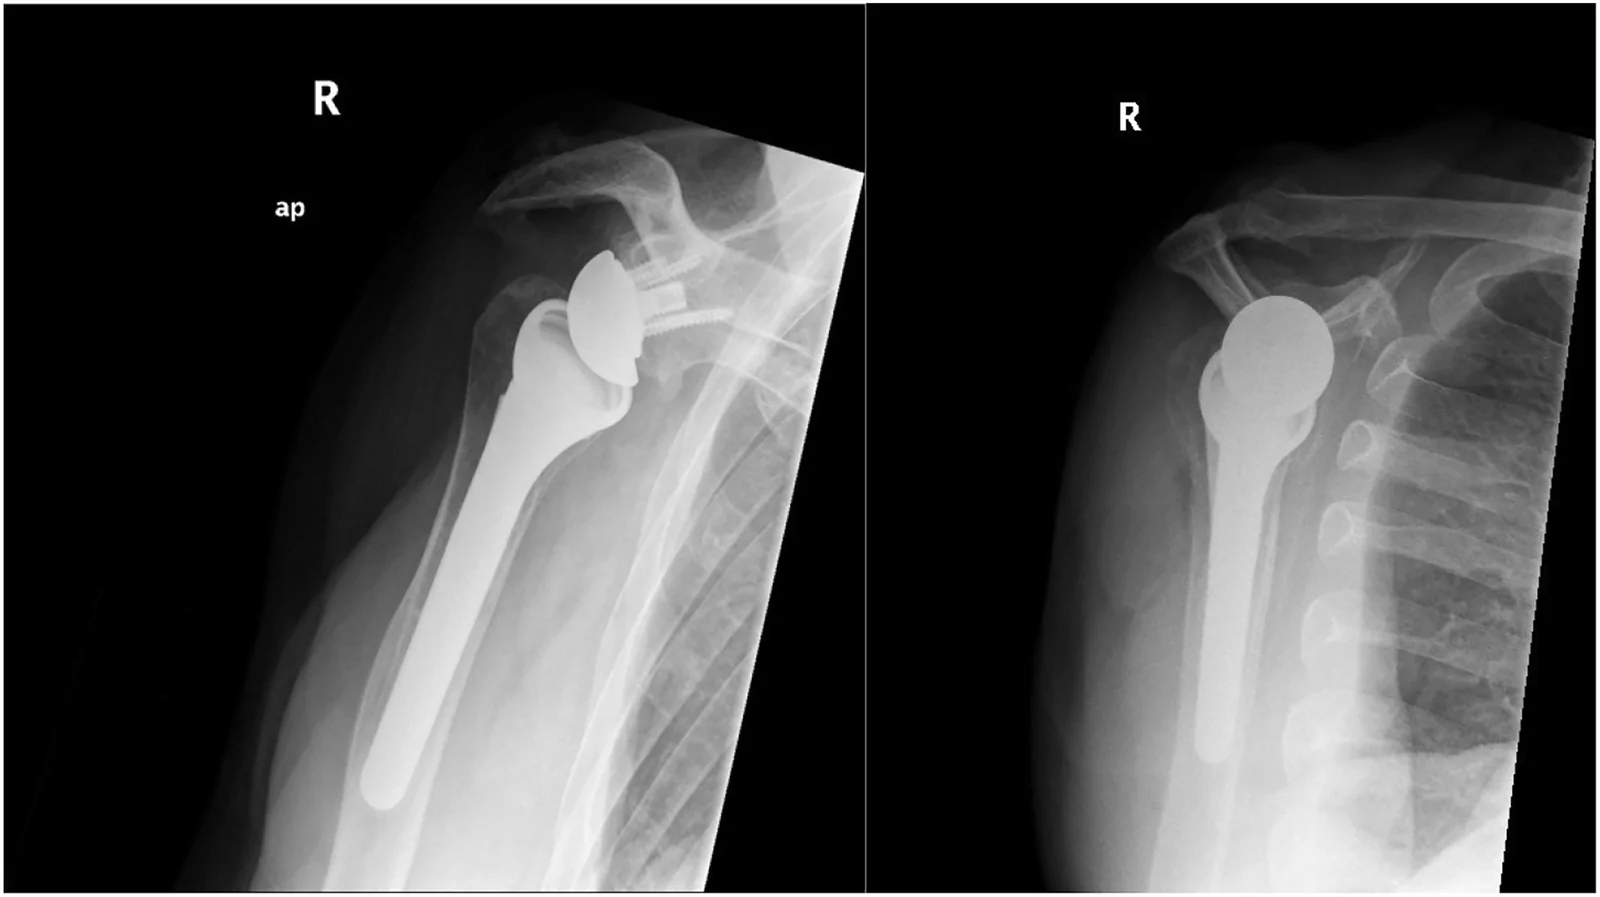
X-rays at 2-year follow-ups show stable implants without any signs of complications.
